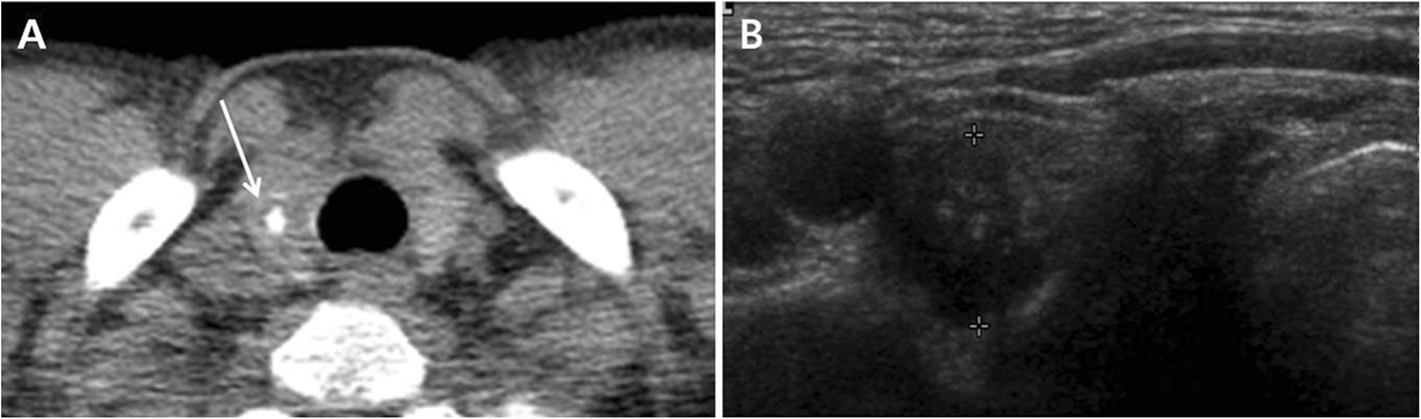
A 60-year-old man with papillary thyroid carcinoma. (A) Low-dose helical CT scan demonstrated a 6 mm densely calcified nodule (arrow) in the right thyroid lobe. (B) Transverse US image of the right thyroid lobe showed a hypoechoic mass (calipers) with internal calcifications.
